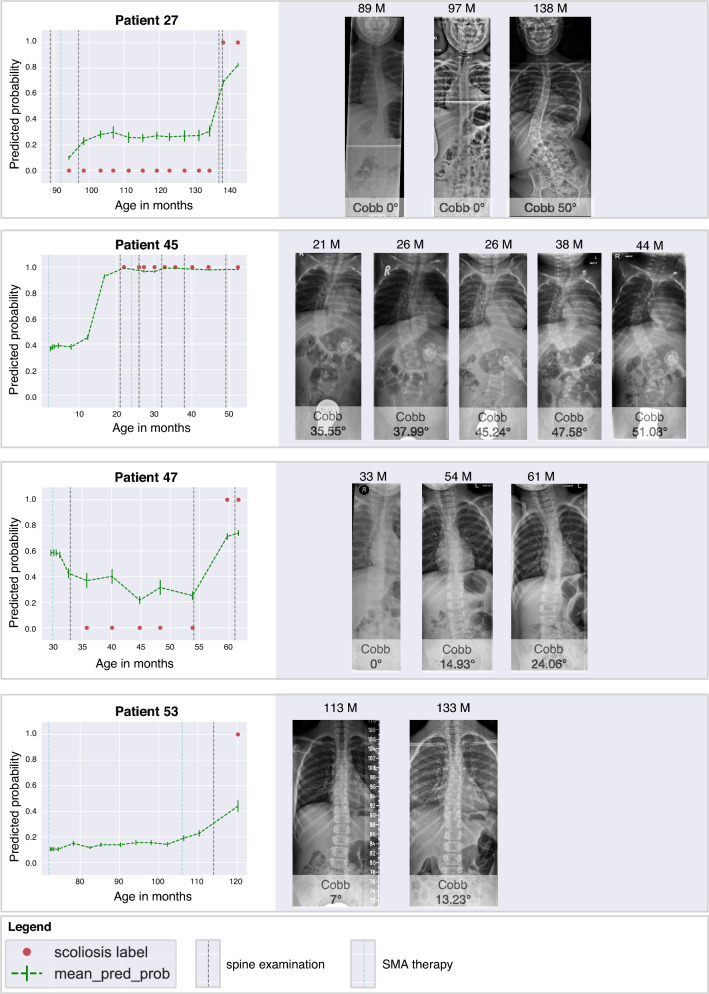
The model’s predicted scoliosis probability (green line) for patients 27, 45, 47, and 53 and their corresponding spine examinations or radiographs taken at the marked time points (grey line). The model was trained on data points corresponding with the scoliosis labels (red dots).
